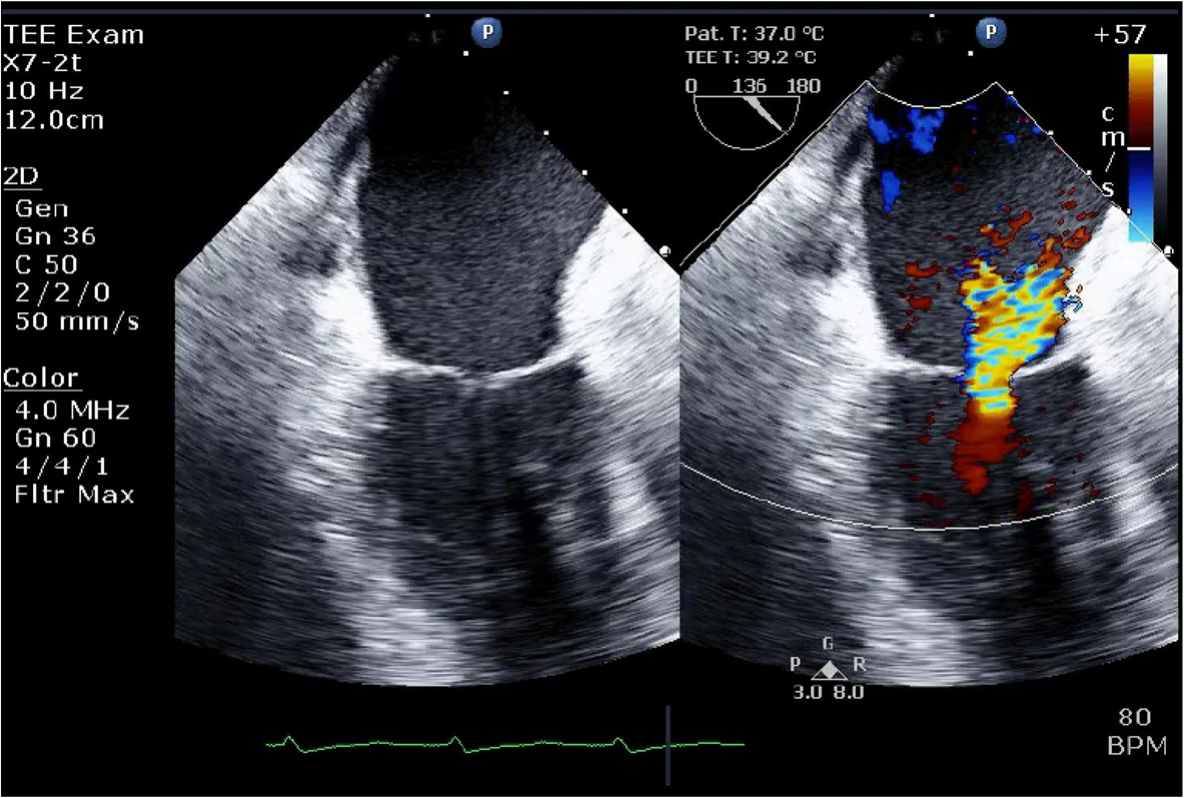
Intraoperative transesophageal echocardiography images after aortic valve surgery, showing a color Doppler image showing severe functional mitral regurgitation caused by left ventricular dilatation
